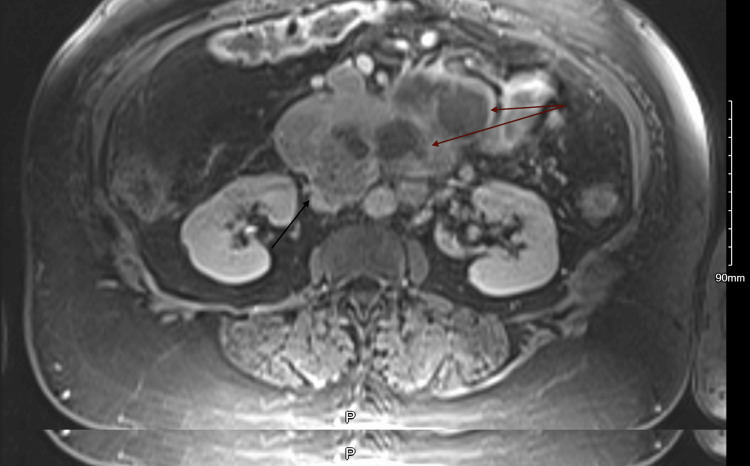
MRI of the abdomen. Black arrow: bulky pancreatic head without a discrete mass. However, the pancreatic head is contiguous with the duodenum without a clear fat plane concerning infiltrating disease. Red long arrows: along the uncinate process of the pancreas, there are ill-defined multiloculated cystic lesions.
